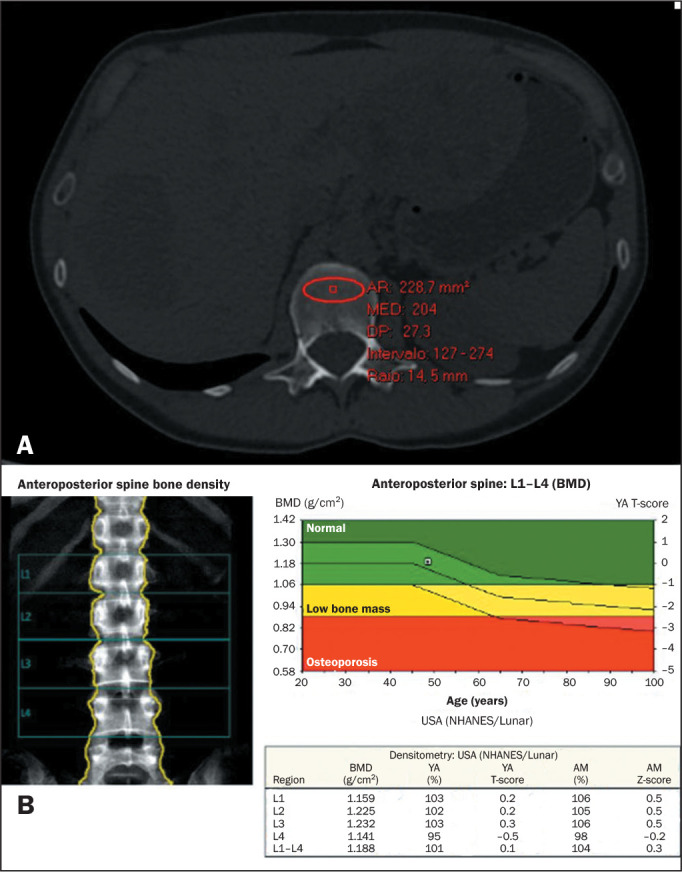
A: Abdominal CT scan with bone window settings, performed on a
